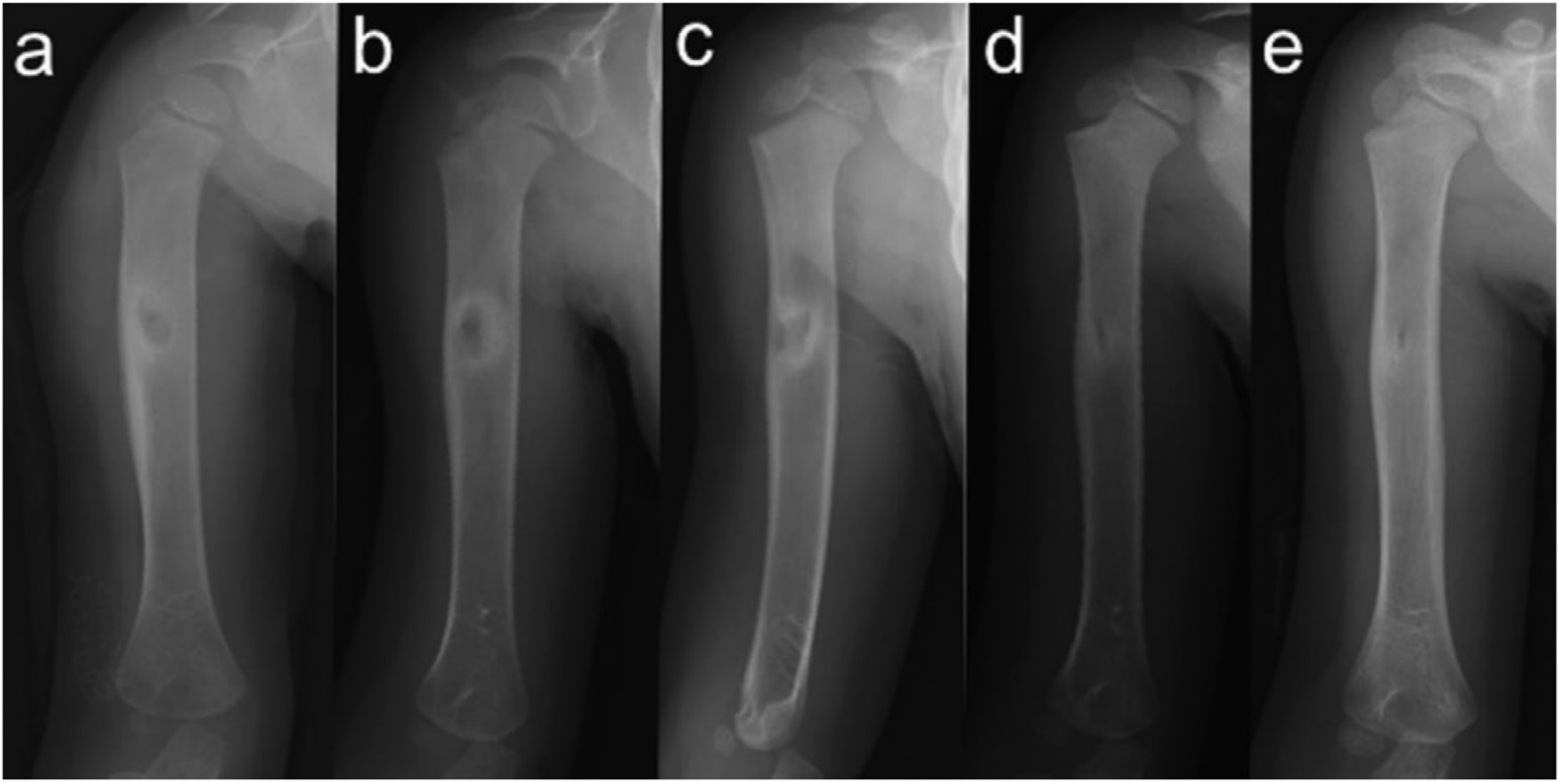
Postoperative anteroposterior radiograph of right humerus taken immediately after surgery (A), 3 months after surgery (B), 6 months after surgery (C), 1 year after surgery (D), and 2 years after surgery (E). The bone lesion resolved over time.
